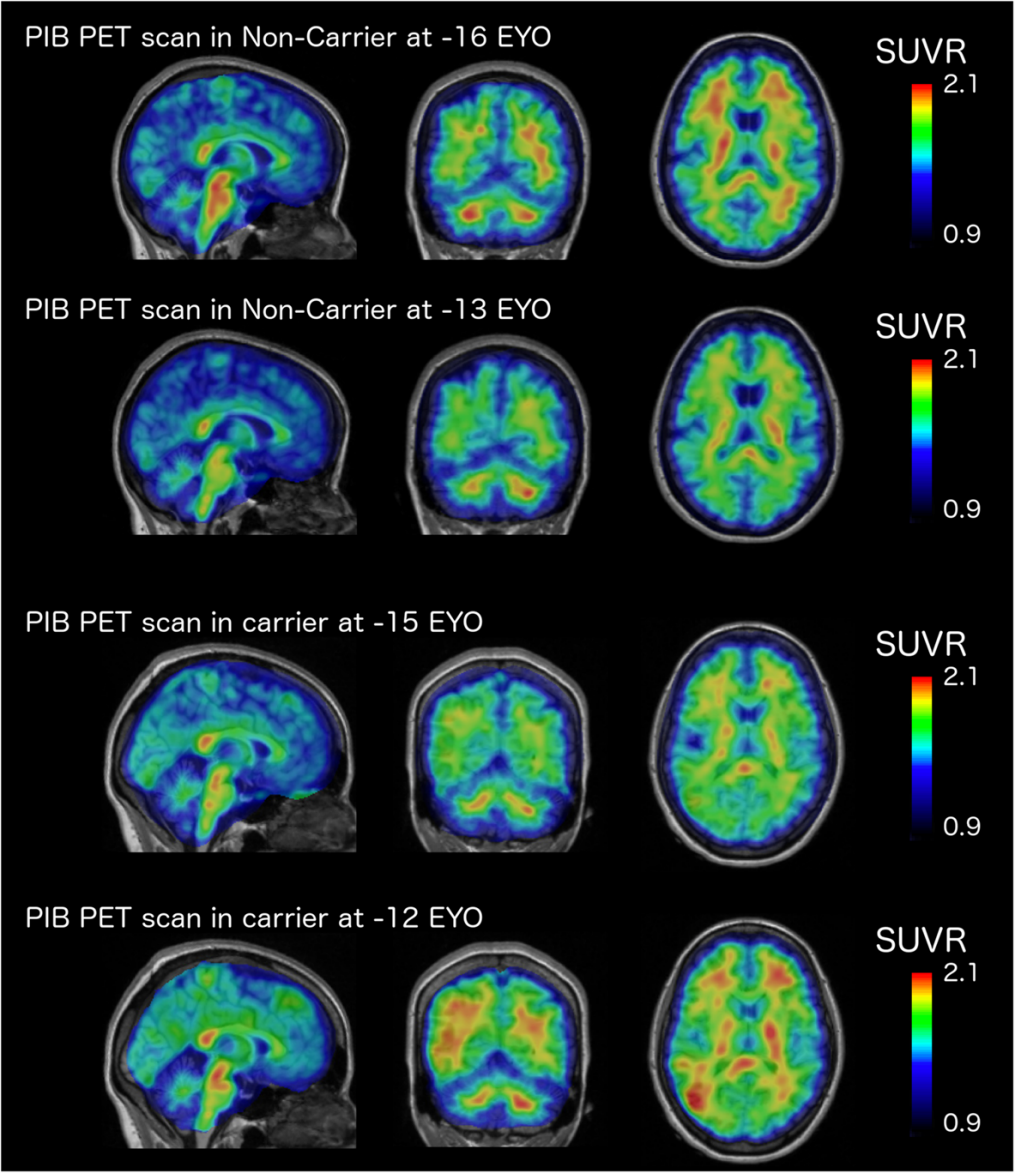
Pittsburgh compound B (PiB) uptake in the brain of a presymptomatic *PSEN1* M84V carrier is consistent with presymptomatic autosomal dominant Alzheimer’s disease mutation carriers. ^11^C-PiB positron emission tomographic scans were performed longitudinally in a *PSEN1* M84V noncarrier and carrier. The color scale for standardized uptake values (SUV) indicate red (high), yellow (medium), and blue (low) PiB retention. *EYO* Estimated years of onset
